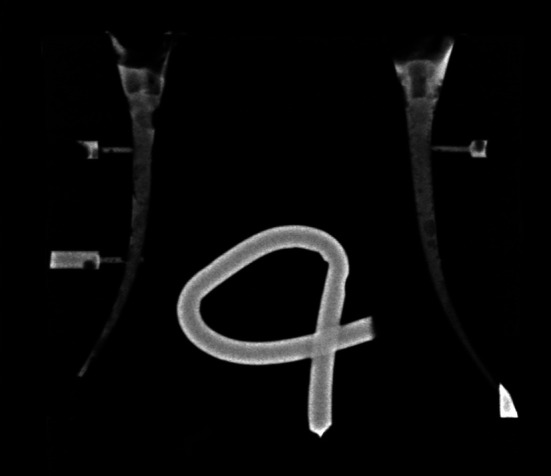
Example of evaluation method; x-ray of A4 and 4B canals, apical lateral canal 4B was considered unfilled.
